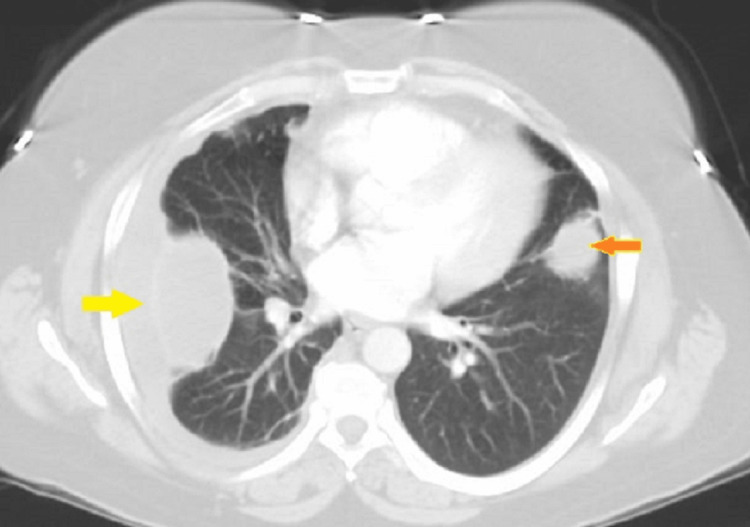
CT of the chest showing empyema and lung mass in the right lung (yellow arrow) and left lung nodule (orange arrow with yellow border).
